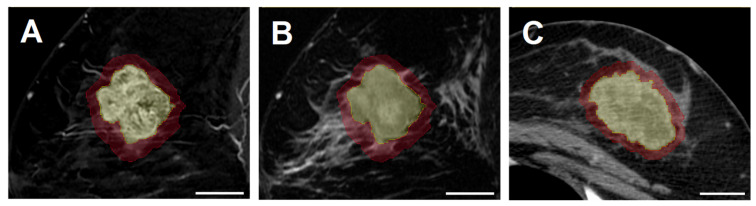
Examples of intratumoral (yellow) and peritumoral (red) segmentations on (A) contrast-enhanced T1-weighted MRI (CE-T1), (B) T2-weighted MRI, and (C) contrast-enhanced CT images. All images are from the same patient, with (A,B) displayed in the sagittal plane and (C) in the axial plane. White scale bars at the bottom right of each image correspond to 2.5 cm.
